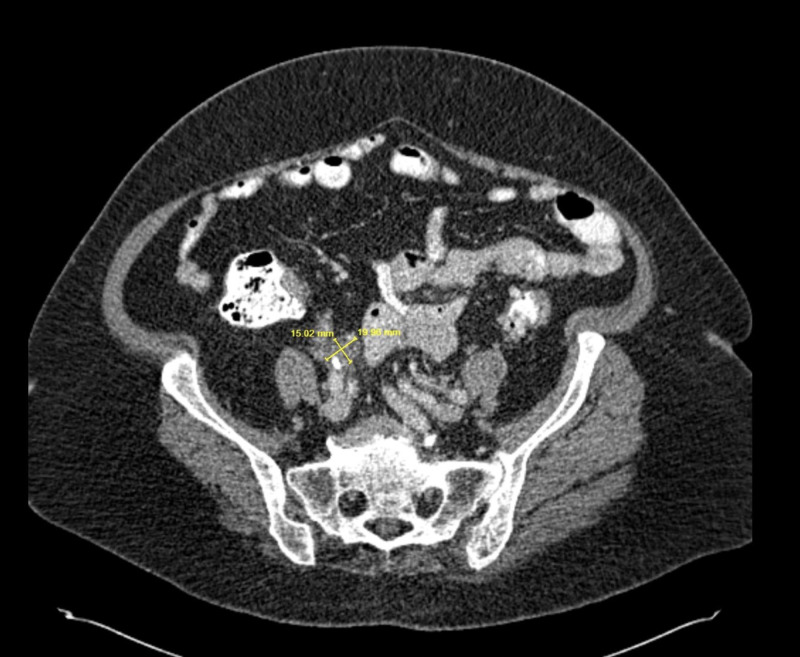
CT of the abdomen W/contrast showing decrease in soft tissue mass just proximal to the iliac bifurcation along the right common iliac artery.
